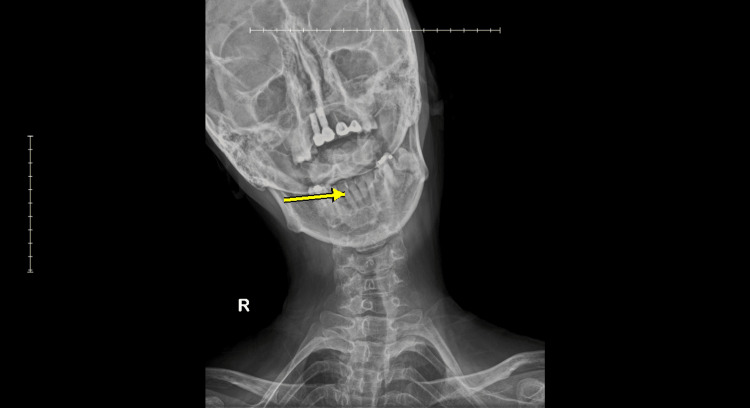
Anteroposterior open-mouth radiograph in right lateral flexion. Arrow indicates the upper cervical region.
